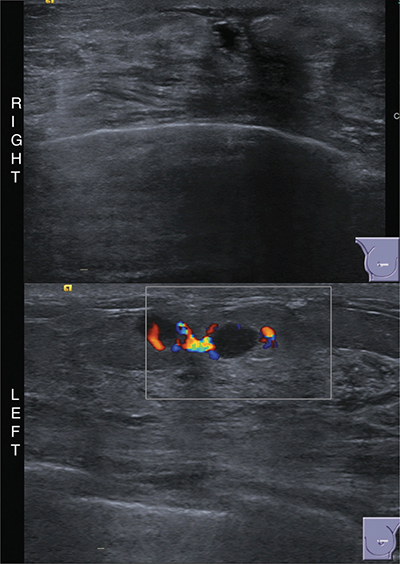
Breast ultrasound with right postsurgical changes, left reveals clustered vascularized masses.
